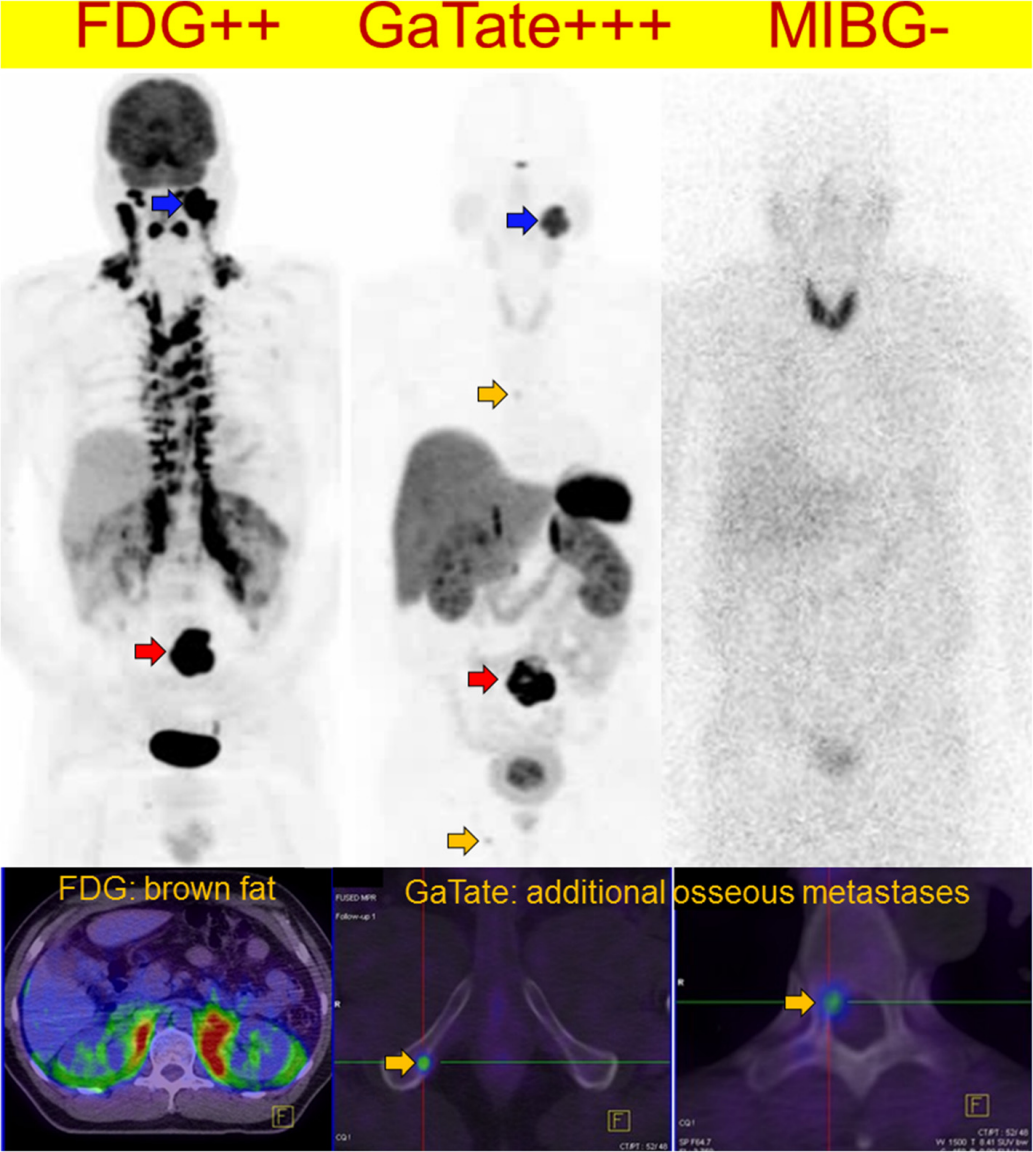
Patients with *SDHB*-related metastatic PGL showing extensive brown adipose tissue above and below the diaphragm on F-18 FDG PET/CT due to high circulating catecholamines (serum normetadrenaline 18 000 pmol/L, normal <900). F-18 FDG MIP (*left*) and axial fused PET/CT (*bottom*) and MIBG (*right*). The peri-nephric and, to a lesser extent central para-vertebral distribution is characteristic of PCC/PGL-related brown fat activation. Ga-68 DOTATATE (*middle*) clearly demonstrated an Organ of Zuckerkandl primary and several osseous metastases (*botttom middle* and *bottom right*), and is not subject to this physiologic artefact. As well as masking potential primary tumour sites along the sympathetic chain, the “sink effect” in brown fat limits availability of tracer for uptake in tumour sites
